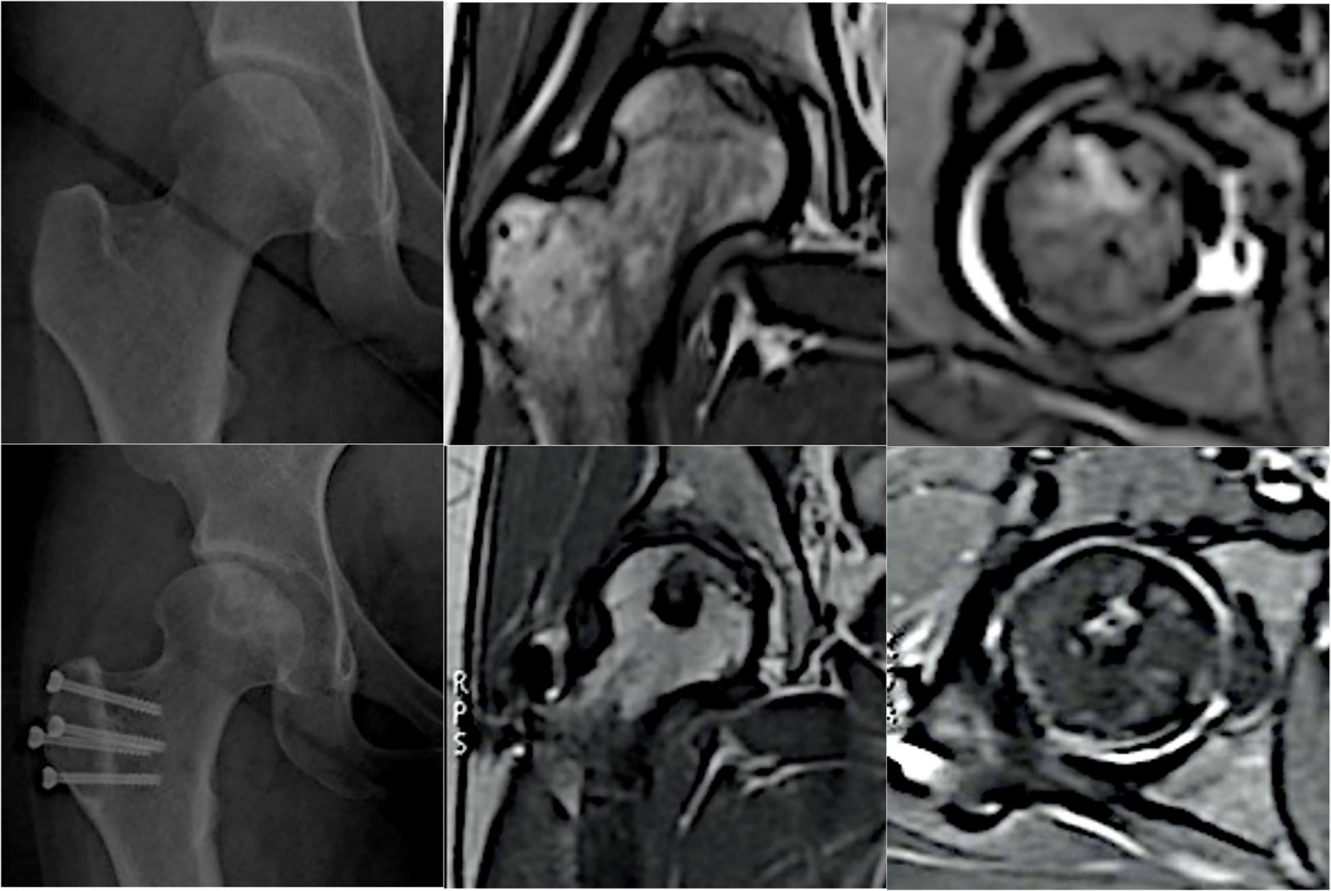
The anteroposterior images indicated the decent integration of the autograft and the femoral head had not collapsed. The magnetic resonance images showed the autograft and round shape of the femoral head
